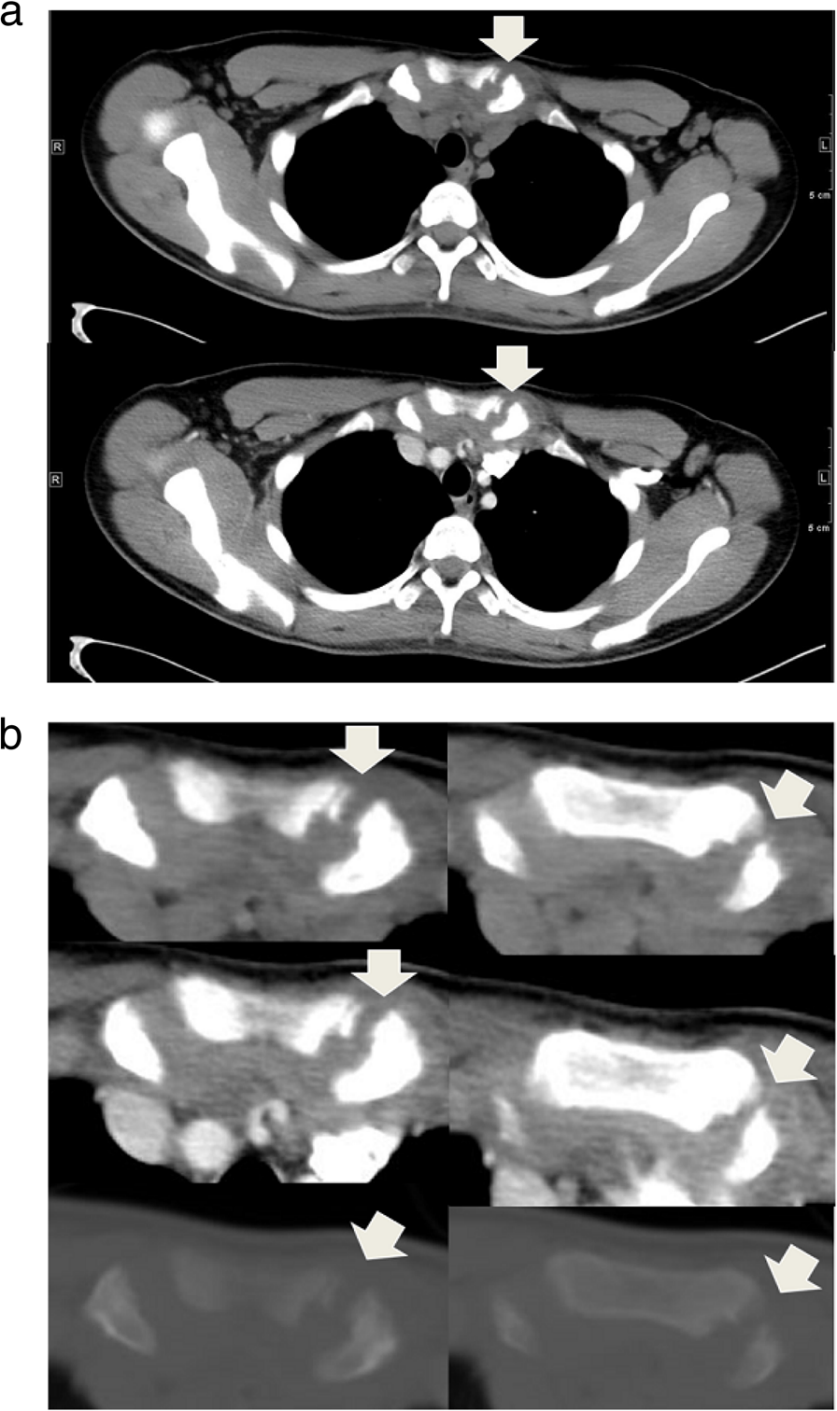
a Enhanced axial computed tomography scan of the sternoclavicular joints (SCJs) shows mild swelling of the left SCJ with thickened synovial lining and mild bone erosion, as well as some turbid fluid collection around the medial end of the clavicle (arrow). b Enhanced zoom-in computed tomography of the sternoclavicular joints (bone window, bottom)
